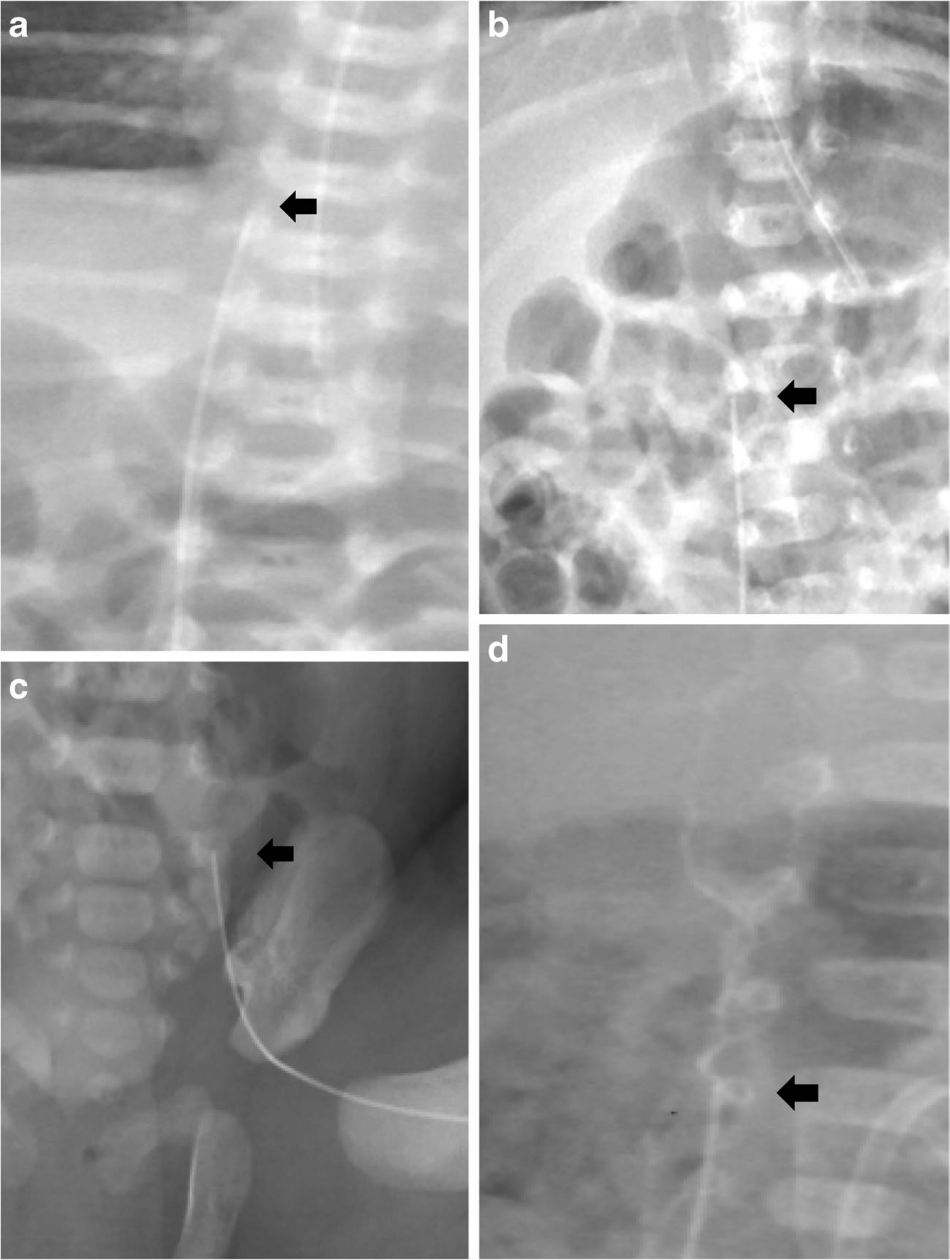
Position of peripherally inserted central catheter (PICC) tips in the lower limb. a A 3-day-old boy. Anteroposterior (AP) chest and abdomen radiograph shows  a PICC tip in a central ideal position—catheter tip between the 8th and the 12th thoracic vertebrae (*arrow*), which corresponds to the inferior vena cava. b A 4-week-old girl. AP chest and abdomen radiograph shows a PICC tip in a central accept position: catheter tip between the 1st and the 3rd lumbar vertebrae (*arrow*). c A 6-day-old girl. AP chest and abdomen radiograph shows a PICC tip beyond the 3rd lumbar vertebra (*arrow*). d A 3-day-old boy. AP chest and abdomen radiograph shows a PICC tip in a non-central position with radiopaque agent in the ascending lumbar plexus (*arrow*)
